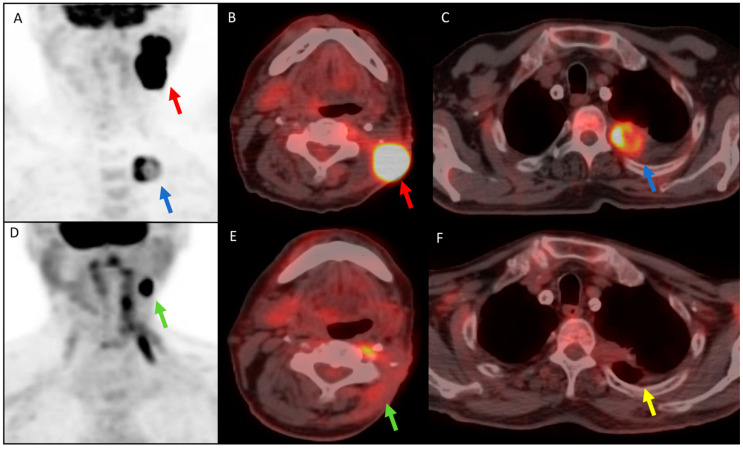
(A–C) ^18^F-FDG PET/CT performed before Nivolumab treatment. (A) Maximum-Intensity Projection (MIP) and (B,C) axial fused images show increased glucose metabolism in the upper left latero-cervical lymph nodes (Maximum Standardized Uptake Value, SUVmax 43.3) (red arrow), in a pulmonary lesion at the left superior lobe (SUVmax 19.1) (blue arrow), and a suspected mediastinal left lymph node at the aortopulmonary window. The disease stage was T1aN1M1. (D–F) Early ^18^F-FDG PET/CT performed 3 months after the start of immunotherapy showed a heterogeneous response in different sites and between functional and morphological co-registered evaluation: on (D) MIP and (E,F) axial fused images, the left latero-cervical lymph node (green arrow) impressively reduced glucose metabolism with a metabolic PR (SUVmax 18.7), while the diameter of lymph node was less than one centimeter on CT-coregistered images (morphological CR). Conversely, the left pulmonary lesion (yellow arrow) showed metabolic CR with an SUVmax <1 but a morphological PR, being still visible on CT-coregistered images although significantly reduced in size.
